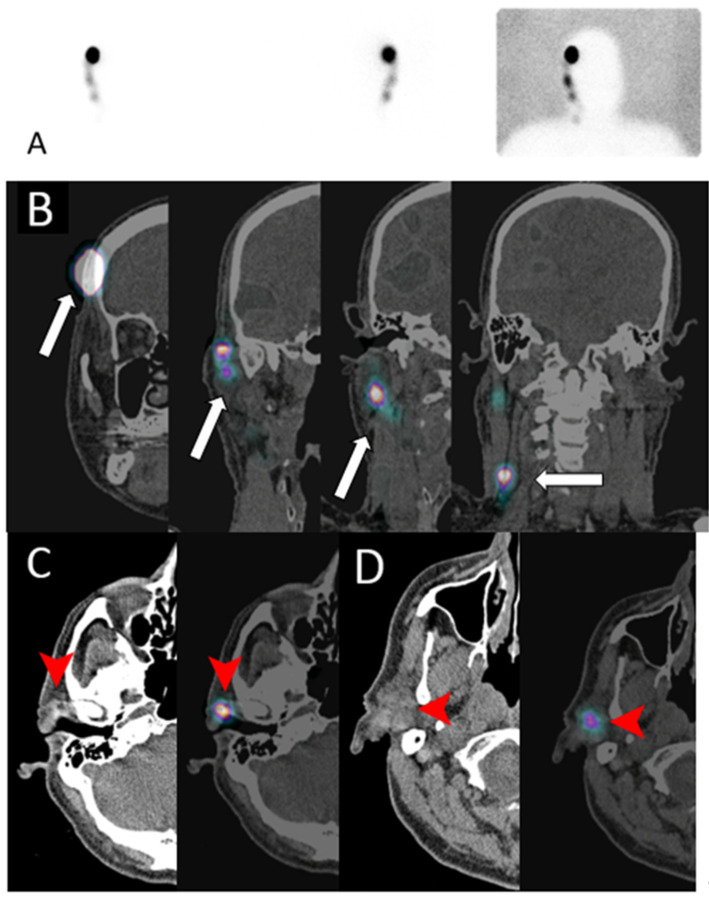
A 68-year-old male with melanoma in the right temporal region who underwent lymphoscintigraphy (A) and complementary SPECT/CT scan in the coronal plane (B); (C,D) show CT (left) and fused SPECT/CT images (right) for sentinel lymph nodes mapping. Intradermal injection of the TC^99m^ sulfur colloid in the right temple (arrow in (B)) shows tracer uptake in the pre-auricular region (arrowhead in (C)) and intra-parotid region (arrowhead in (D)), consistent with sentinel lymph drainage.
